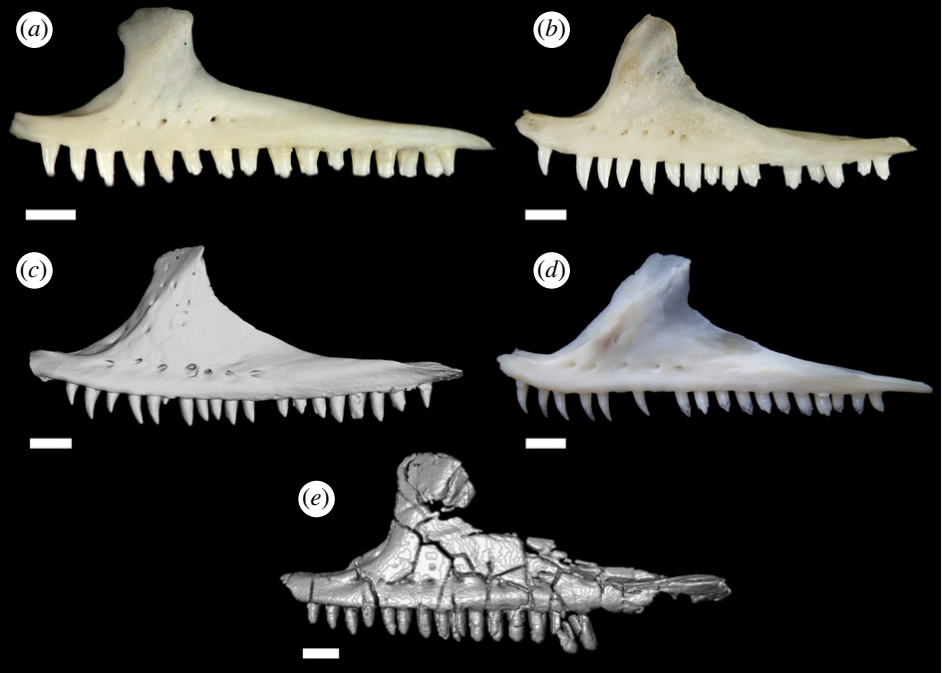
Comparison of maxillae and tooth morphology between extant crotaphytids and *Aciprion formosum* AMNH FR 11400. (*a*) *Crotaphytus collaris* TxVP M-9255; (*b*) *Crotaphytus bicinctores* TxVP M-8612; (*c*) *Gambelia sila* TNHC 95621 (from computed tomography scan); (*d*) *Gambelia wislizenii* TxVP M-8394; (*e*) *Aciprion formosum* AMNH FR 11400. Scale bars = 1 mm.
